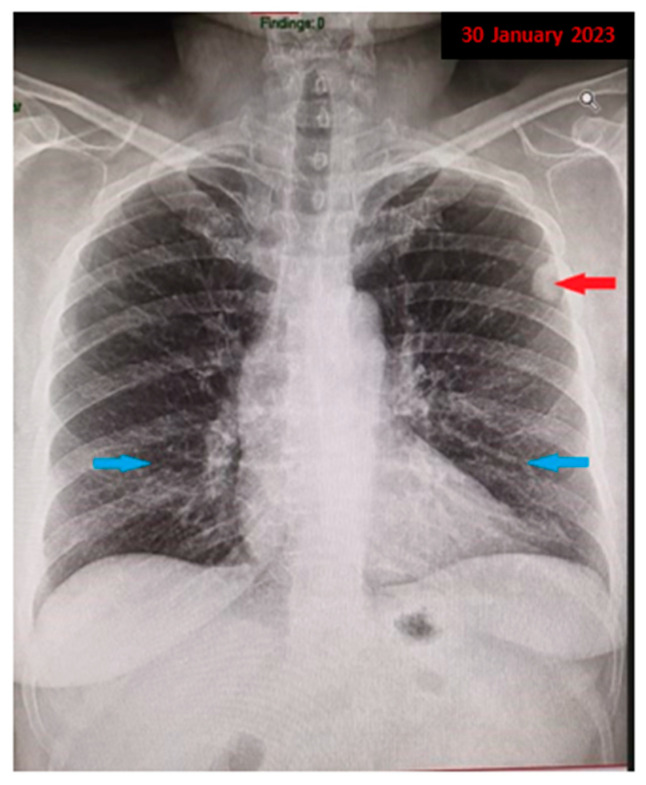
We present the case of a 59-year-old female patient, a former smoker with a history of 15 pack-years, who quit smoking 16 years ago, with prolonged occupational exposure to respiratory irritants (hairdresser for 23 years), who presented to our medical department for a detailed investigation of a lung nodule identified following examinations conducted at a regional medical facility. Her medical history up to this point showed no significant issues, except for a previous diagnosis of renal cysts and chronic treatment with antihypertensive medications (diuretics, angiotensin-converting enzyme inhibitors—ACE inhibitors, and central antihypertensives) for hypertension. In December 2022, she went to a regional medical care unit reporting mixed-type cough, predominantly irritative, with paroxysmal nocturnal exacerbations, moderate-effort dyspnea, and fatigue. Initially, symptomatology was labeled as residual from an acute upper respiratory tract infection. A chest X-ray was performed (Figure 1), which revealed a nodular pulmonary opacity (red arrow) of approximately 20/15 mm, located peripherally in the left upper lobe (LUL), adherent to the pleura, and an accentuated pulmonary interstitial pattern basal, bilaterally (blue arrow). Further, she was recommended to perform a spirometry with a bronchodilator test and additional imaging investigations with a contrast-enhanced chest computed tomography (CT) for accurate diagnostic assessment of the radiologically detected pulmonary nodule.
